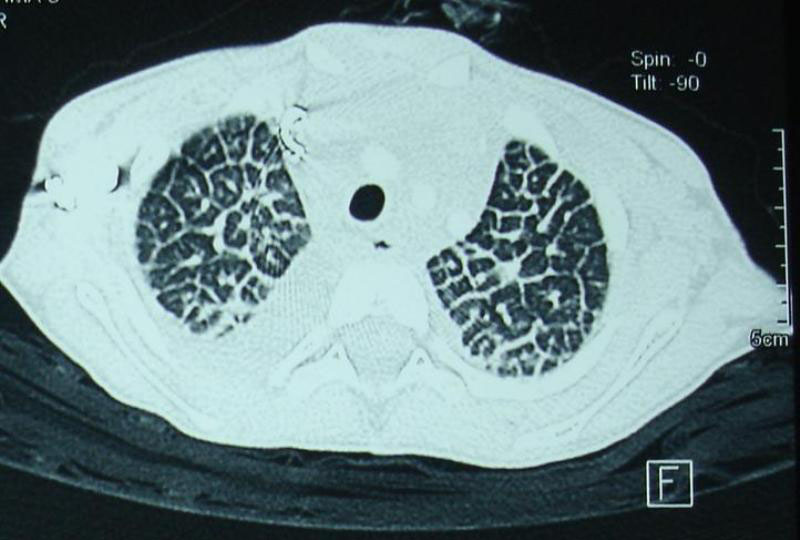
High-resolution computed tomography image of the chest shows thickened interlobular septae with typical polygonal appearance of secondary pulmonary lobule. Small amount of pleural fluid is seen.
